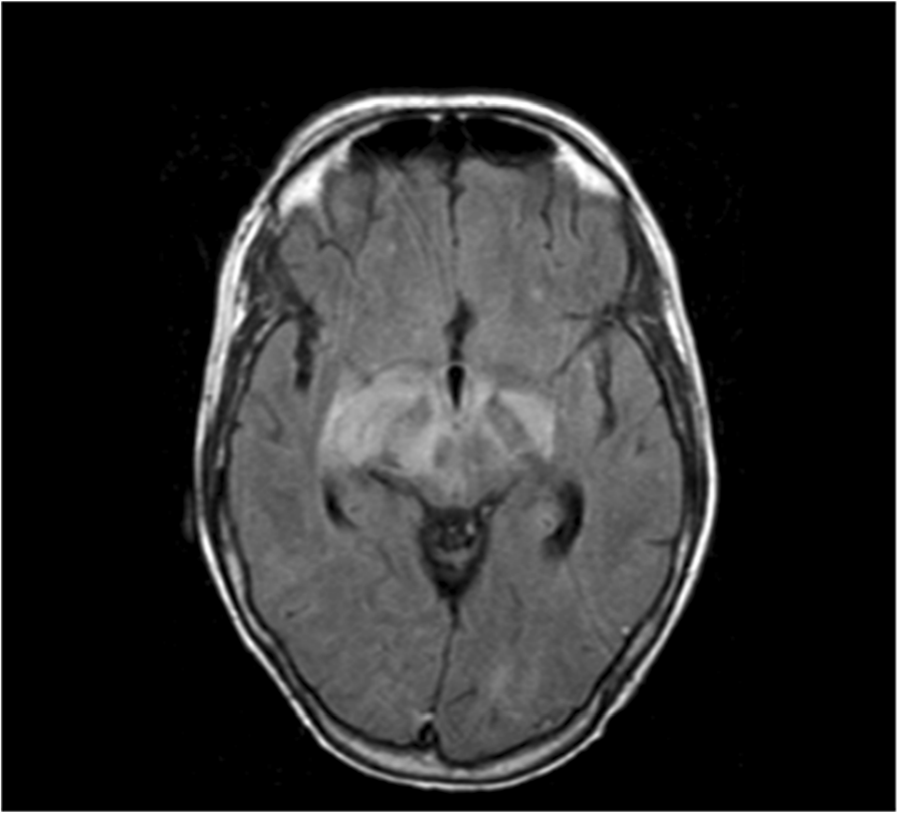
MRI cerebrum (T1), diffuse hyperintensity and swelling, with enhanced ring shaped areas
